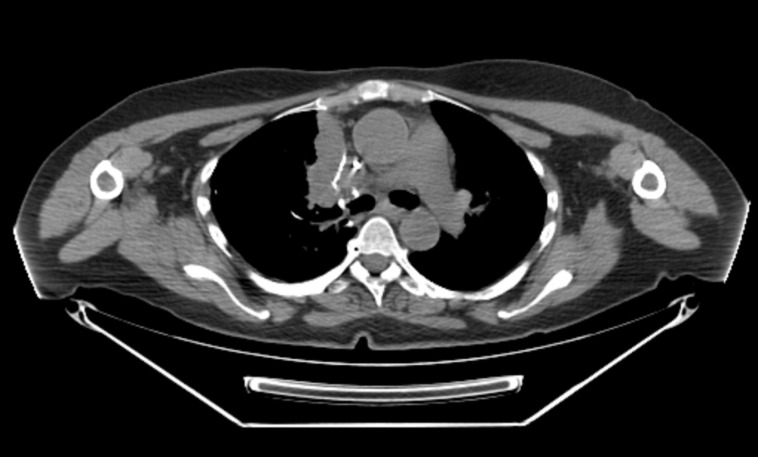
CT scan of the chest in May 2016 A paramediastinal mass, 6.3 x 2.5 cm, is seen.
